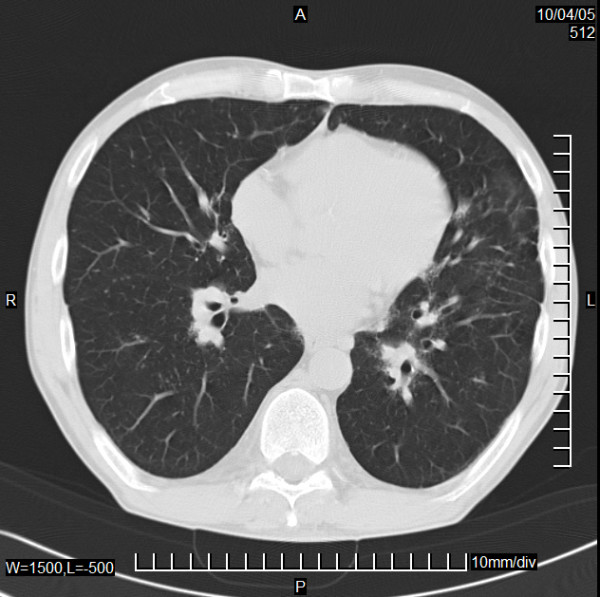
Computed tomography of the chest (II). CT of the chest: Nodular and reticular interstitial lung involvement.
